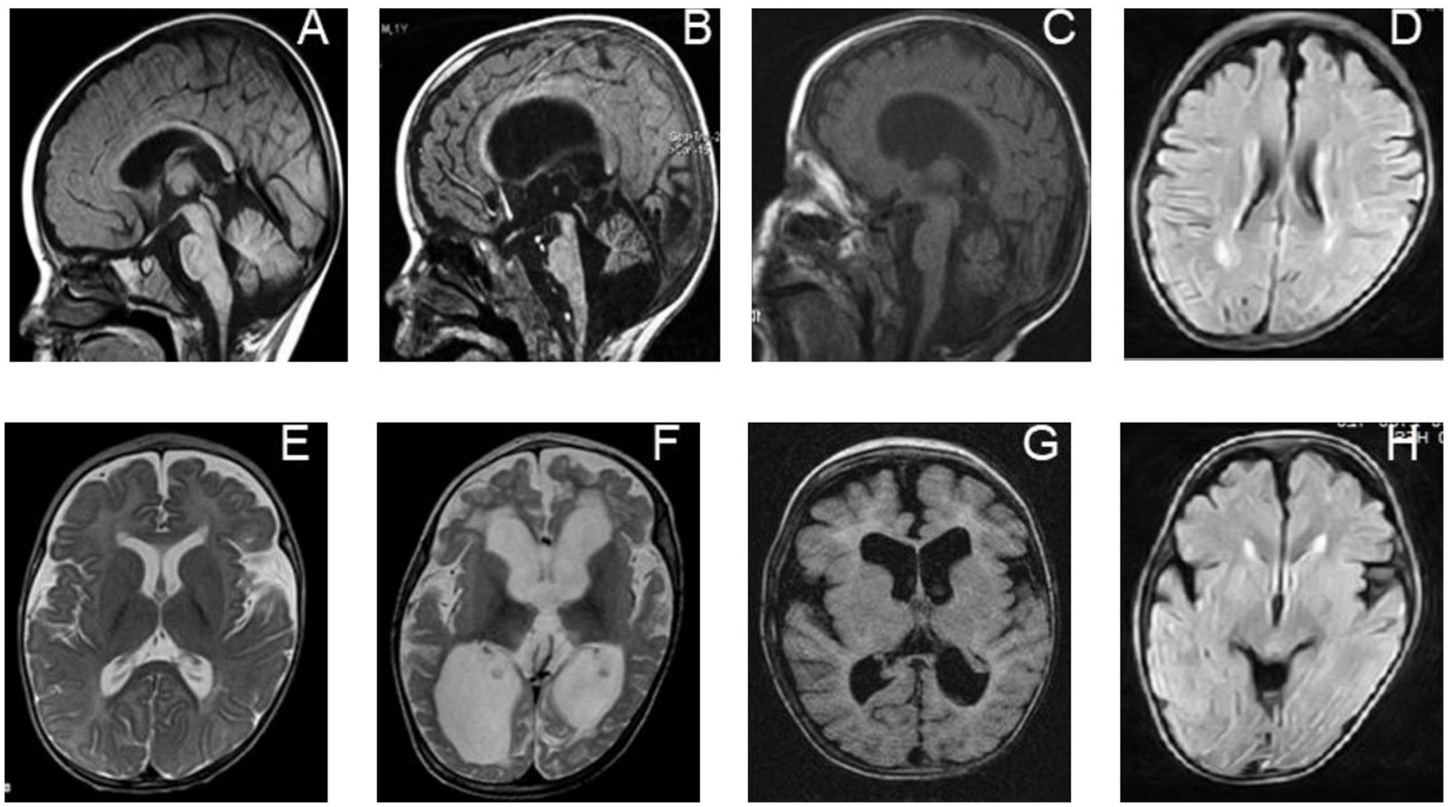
Neuroradiological features of individuals with biallelic *PRUNE1* mutations. Sagittal T1-weighted images of a *PRUNE1*-affected patient performed at 6 months of age (A) and 16 months of age (B) show progressive global brain atrophy, but more specifically evidence of cerebellar and brainstem atrophy, which is out of proportion to the cerebral atrophy. (E,F) Axial T2-weighted images performed at 6 months of age (E) and 16 months of age (F) in the same child, showing progressive diffuse white matter abnormalities along with progressive brain atrophy. (C) Axial inversion recovery and (G) sagittal T1-weighted MRI sequences performed in the patient at 24 months of age show generalized brain volume loss, but with specific evidence of cerebellar atrophy and a diffuse white matter signal abnormality as was seen in her sibling (D,H).
